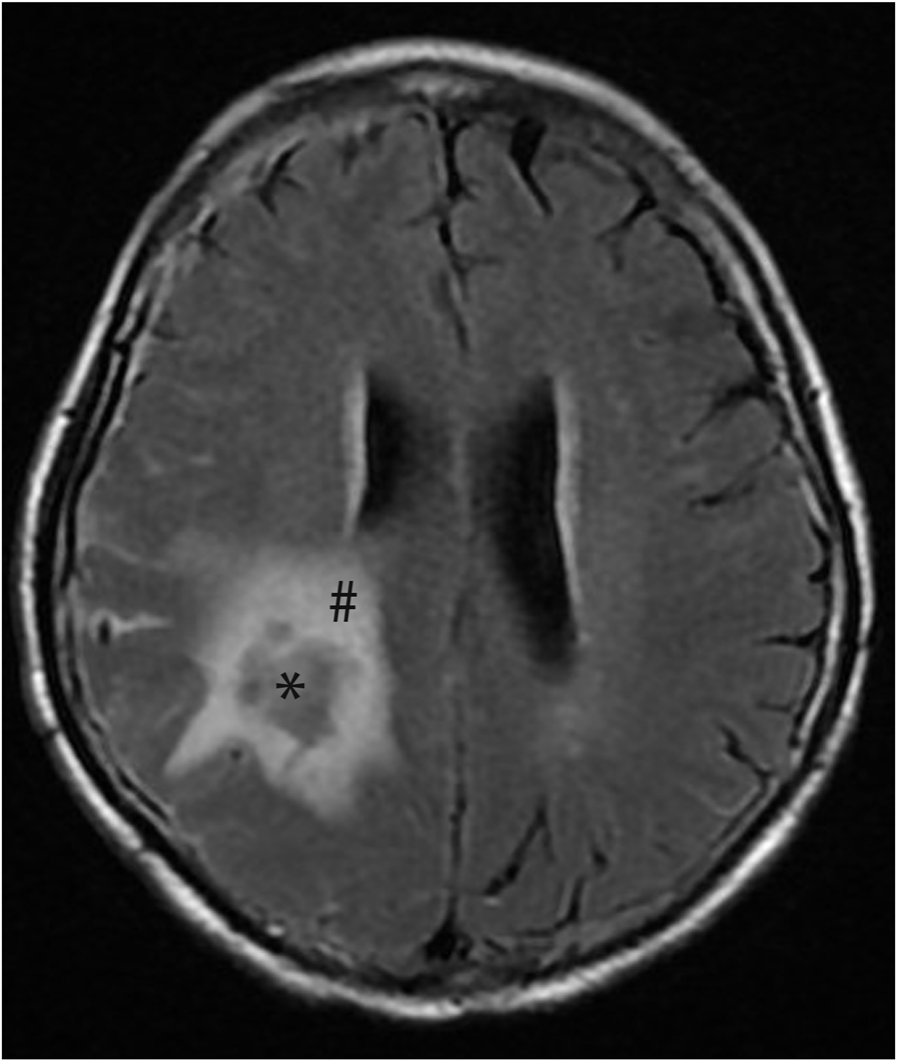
Flair magnetic resonance imaging in a patient at day 1 after ICH. Note the marked edema (^#^) surrounding the hematoma (*).
